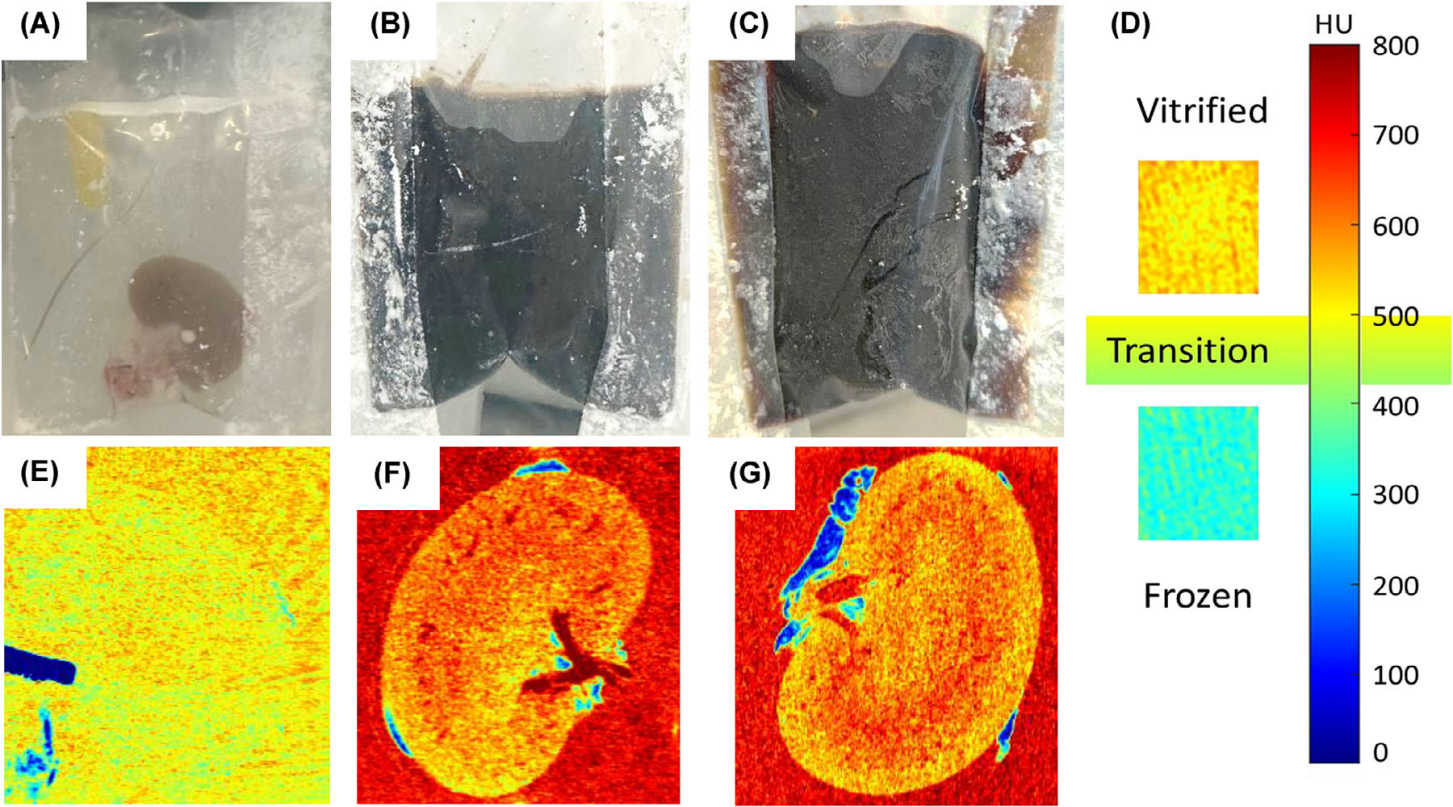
Photographs and Micro‐computed tomography of vitrified kidneys with and without IONP loading. Top row: photographs of polyethylene bags containing vitrified kidneys loaded with a) VMP (CPA control), b) Ultracentrifuged sIONP in VMP, and c) TFF‐purified sIONP in VMP, d) Pseudo color scale represents micro‐CT image radiodensity in Hounsfield units (HU). Bottom row: e,f,g) micro‐CT pseudo color images of vitrified kidneys in the top row (a), (b), and (c), respectively. The HU scale differentiates between frozen and vitrified kidneys based on attenuation. Low attenuation of ˂ 400 HU is indicative of a frozen kidney, while > 500 HU indicates a vitrified kidney^[^
